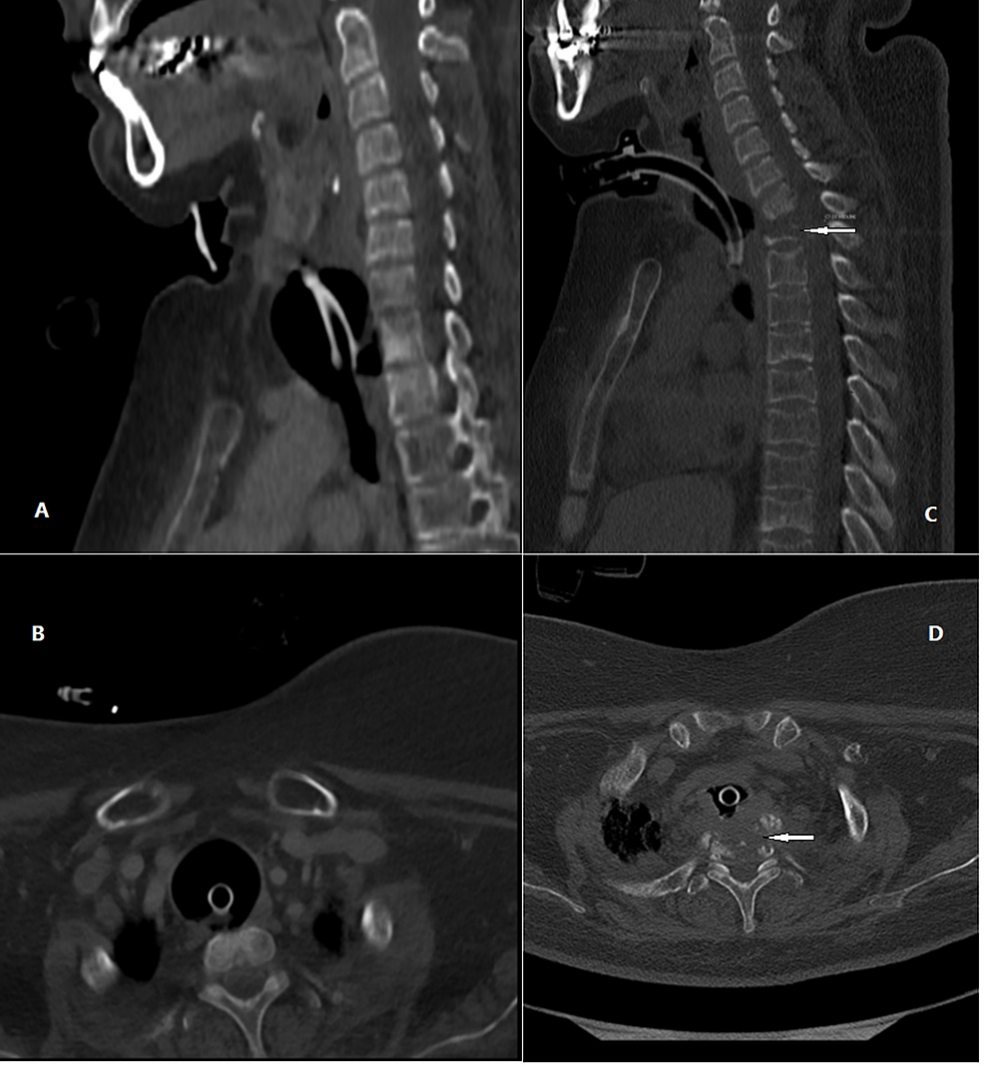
Neck CT images (A) Sagittal view neck CT image. (B) Axial view neck CT image. The neck CT scan was performed five months before vertebral destruction. CT scan demonstrates the normal shape of vertebral bodies with mild reactive sclerosis and minimal erosion in the anterior aspect of Th1. (C) Sagittal view neck CT image. (D) Axial view neck CT image. The neck CT scan was performed during hospitalization. CT scan demonstrates the destruction of two vertebral bodies, C7 and Th1 (arrows). CT, computed tomography.
